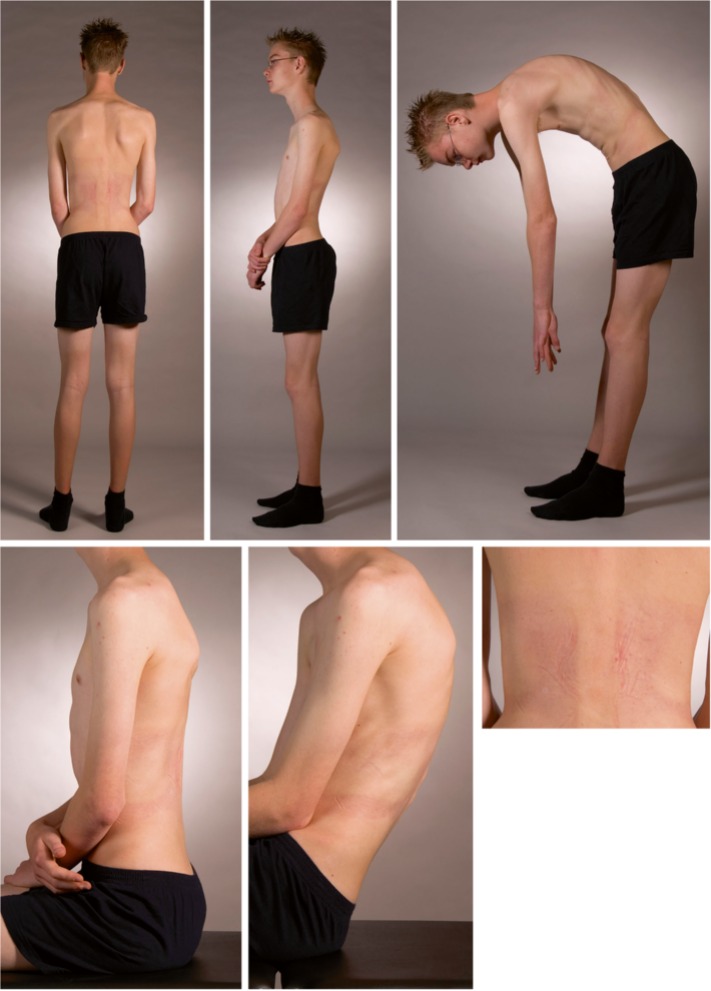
A fifteen year old boy with progressive Scheuermann kyphosis and serious back pain. Late referral but first treated with physical therapy between first and second X-ray. Wedging and Schmorl’s nodi mainly in the thoracolumbar area (Cobb Th10-L2: 20°). Picture taken after the first night wearing a TLI brace: Clockwise: Left: standing, some scapular asymmetry is present. Middle: lateral view while active standing upright. Right: the contracted neuromuscular system while bending forward. Note the impossibility to keep the legs vertical, get the lumbar spine horizontal and the visible tight hamstrings. Flexion occurs in the upper spine, not around the hips. Under right : the skin of his back after the first night of wearing a TLI brace. The main pressure is on the paraspinal musculature with the apex at the thoracolumbar area at the same level as the elbow pits. There is no pressure mark on the processi spinosi or the lower ribs. In the middle: sitting with straight legs and left sitting on the edge of the couch. The thoracolumbar kyphosis redresses itself somewhat. TLI will maintain this continuously with progressive reduction.
